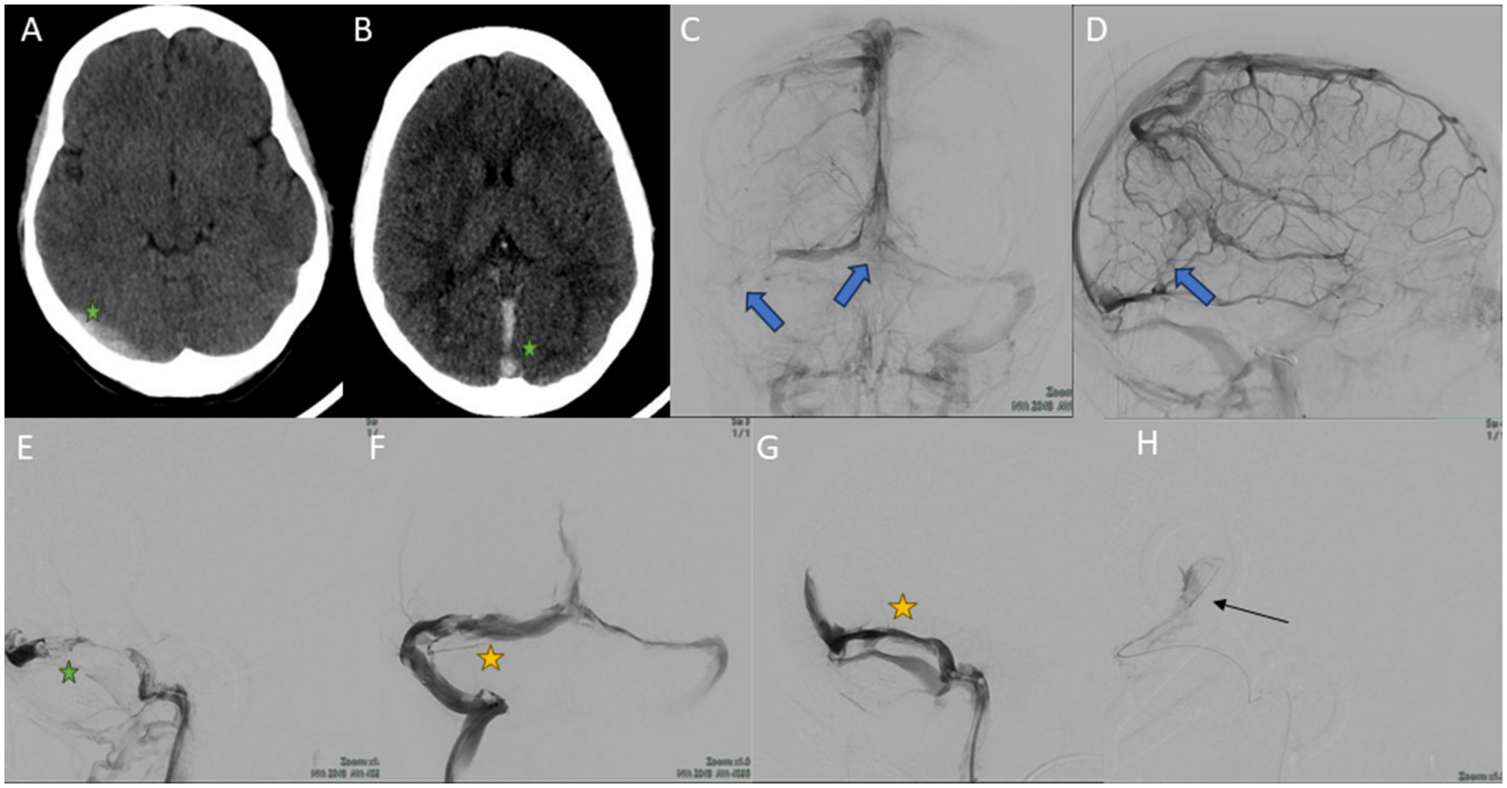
Images obtained during the course of case 2. (A, B) Cerebral CT scan without intravenous contrast. There is evidence of hyperdensity at the right transverse venous sinus, rectus sinus and superior sagittal sinus (green stars) due to acute thrombosis. (C, D) Selective right internal carotid arteriography in venous phase, anteroposterior (C) and lateral (D) view. Multiple repletion defects are evident in both transverse sinuses [blue arrow in (C)], torcula [blue arrow in (C)], superior sagittal sinus, rectus sinus and deep cerebral venous system [blue arrow in (D)], secondary to thrombosis. (E–H) Cerebral venography obtained by left internal jugular access, MT. (E) Lateral view. Occlusion of the right transverse sinus (green star). (F) Anteroposterior view. Recanalization of the right transverse sinus after endovascular treatment (yellow star). (G) Lateral view. Occlusion of the rectus sinus is seen (yellow star). (H) Lateral image view. Microcatheter (black arrow) is advanced through the rectus sinus prior to recanalization by stent retriever with TigerXL^®^ device (not shown in the image).
